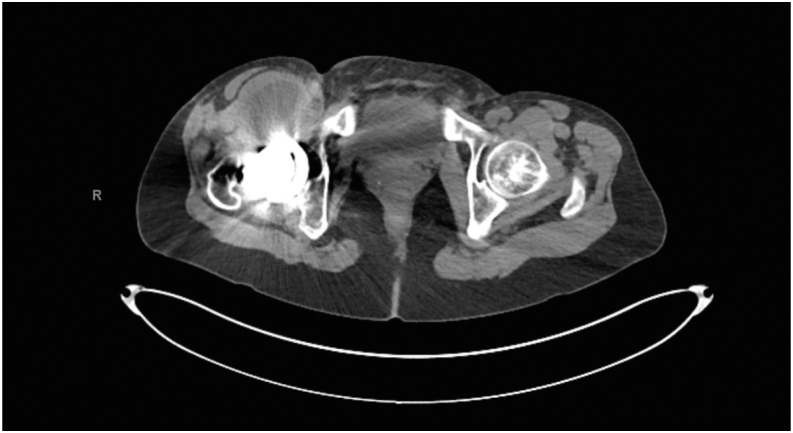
Axial view of the pelvis. CT scan demonstrating acetabular component overhang and impingement of the iliopsoas over the femoral head.
